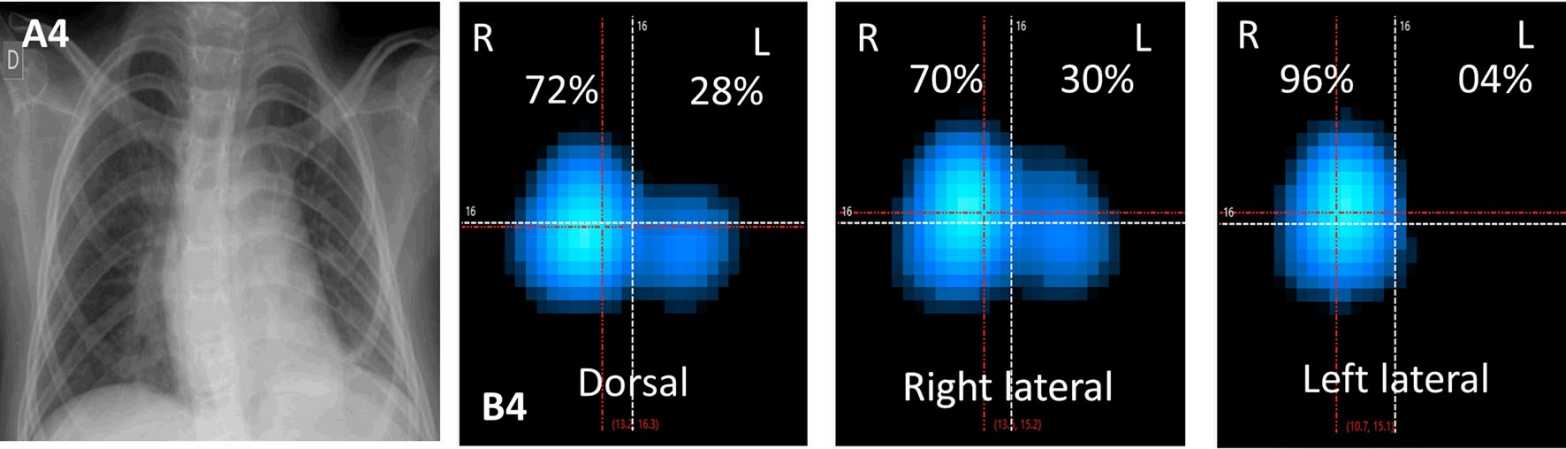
The figure shows chest radiograph images and EIT images of patient 4, a four years-old girl, in 3 different decubitus: dorsal, right lateral and left lateral. The patient was using NIV, through a face mask, with an inspiratory pressure of 20 cmH_2_O and a positive end-expiratory pressure of 8 cmH_2_O. The radiograph image A4 show clamping of costal arches and elevation of the left diaphragmatic dome suggestive of atelectasis. The EIT, other three panels, shows the distribution of ventilation according to the patient's position. The color scale of the EIT corresponds to the tidal volume variation present at the different ROIs; dark blue corresponds to a lower ventilation variation, and white corresponds to high tidal volumes. In the supine position (B4), a decrease in ventilation on the left lung is observed, compatible with the radiological image. When the right decubitus position was adopted, a ventilation increase in the dependent right lung was observed, consistent with the physiological response. When the left lung was in the dependent position, left lateral decubitus, almost no ventilation in this lung was detected (4%). Most likely, the action of gravity leads to the dynamic closure of small airways, limiting the flow, and preventing ventilation
